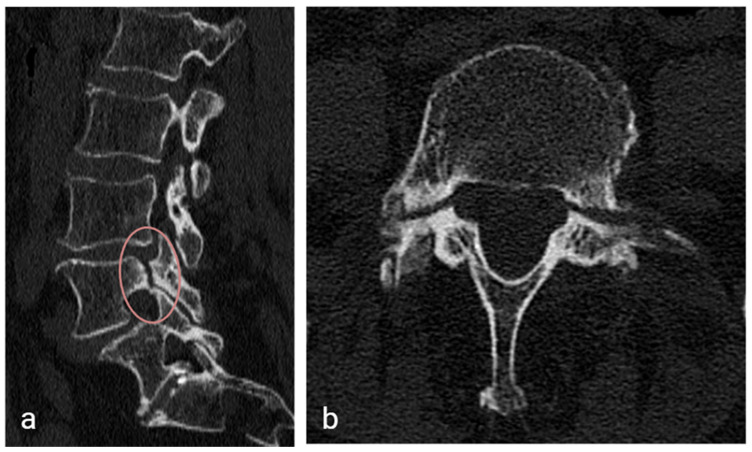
CT images showing bilateral L4 pedicle stress fractures with short oblique fracture orientation at L4–L5 and pedicle sclerosis. (a) Sagittal view. (b) Axial view.
